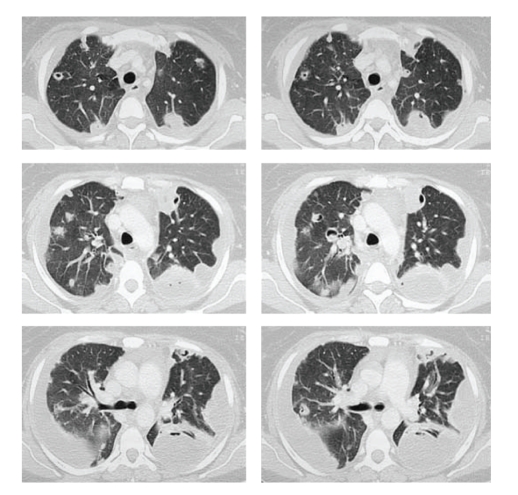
CT scan revealed nodular opacities and diffuse
